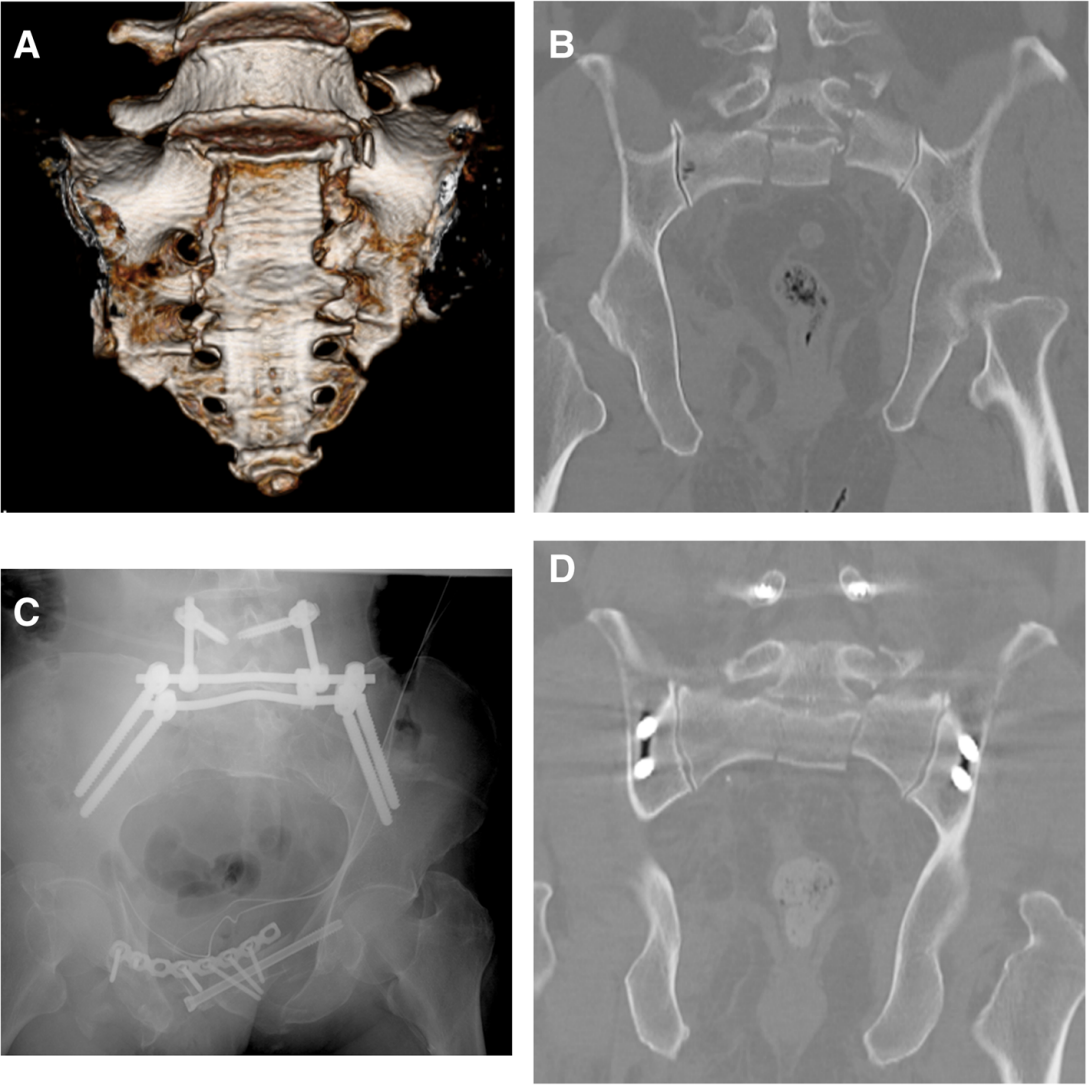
Case 15: a 45-year-old male who sustained an unstable AO type C2 pelvic ring fracture in a traffic accident, including a bilateral fracture of the Denis zone II of the sacrum and a 15-mm vertical dislocation (cranial direction). a Preoperative three-dimensional computed tomography (CT), showing the H-shaped sacral fracture. b The 15-mm vertical dislocation (cranial direction) is observable on the preoperative coronal plane CT. c Postoperative radiographs, showing the crab-shaped fixation. d Postoperative CT showing a residual 7.5-mm vertical dislocation (cranial direction)
